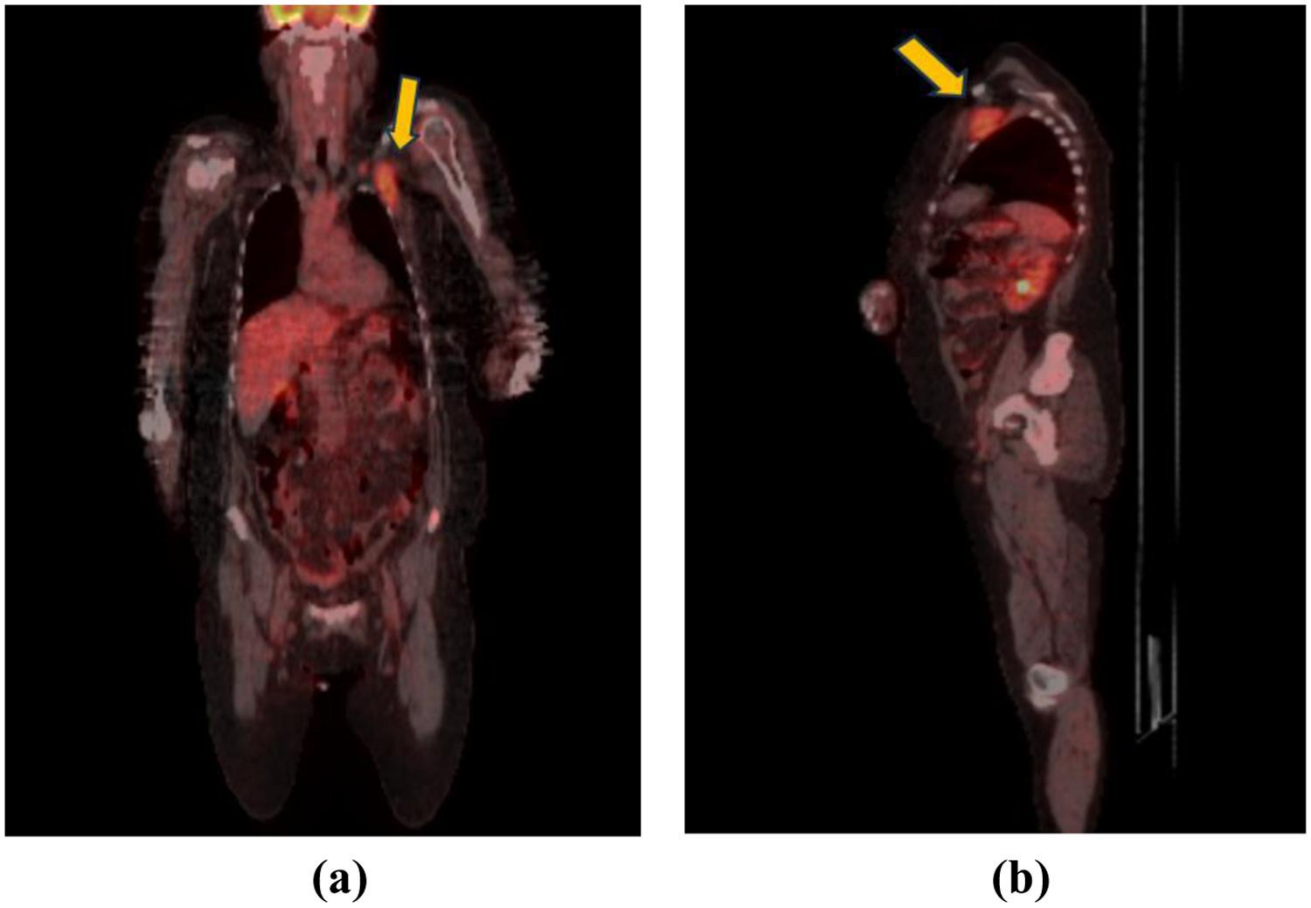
(a,b) PET—CT image—hypersensitive interpectoral formation.
